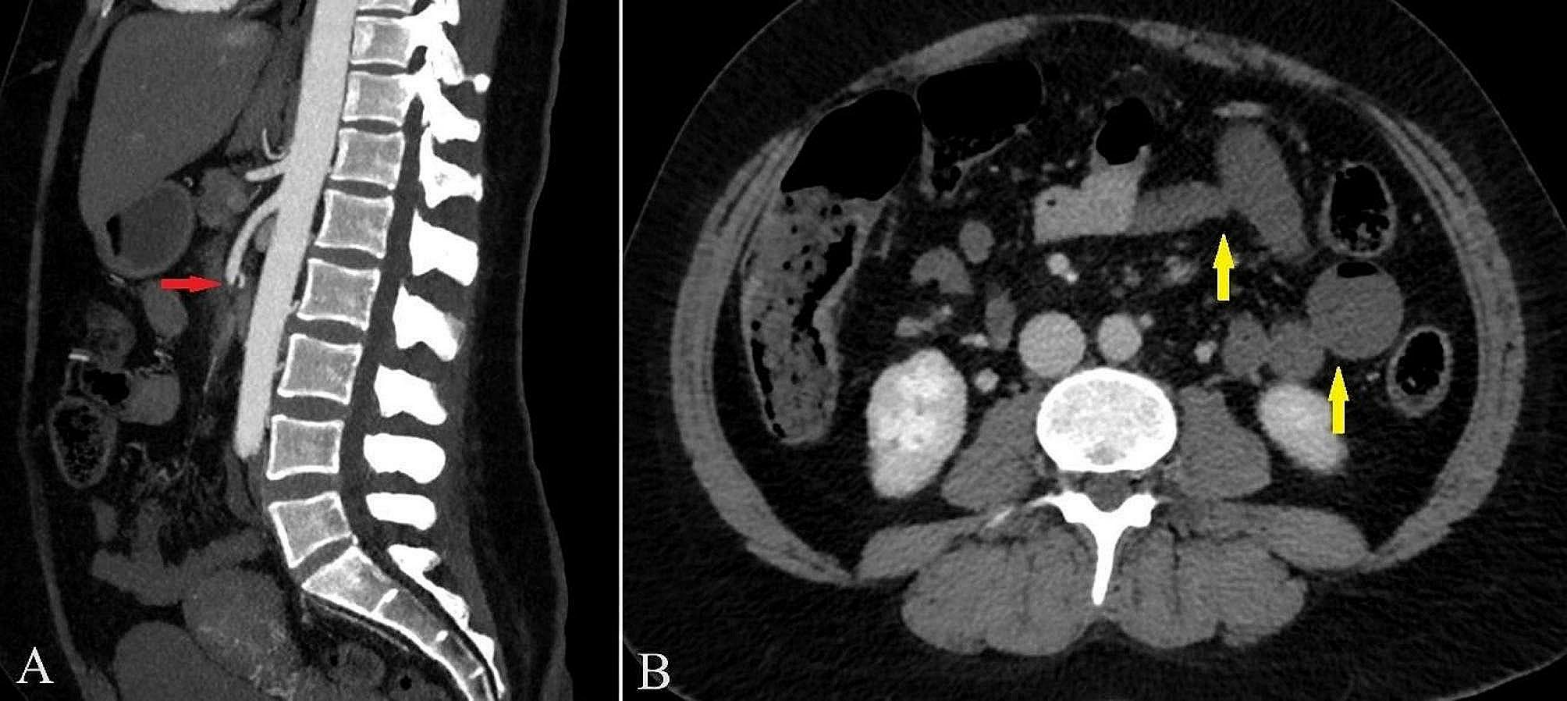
(A) Filling defect within the superior mesenteric artery approximately 4 cm distal to its origin consistent with occlusion (red arrow) on the sagittal maximum intensity projection (MIP) reconstruction CTA images. (B) The occlusion resulted in (B) perfusion changes: lack of enhancement and mild dilatation of the jejunum (yellow arrows) on the portal venous phase (Reference: Semmelweis University – Medical Imaging Centre)
